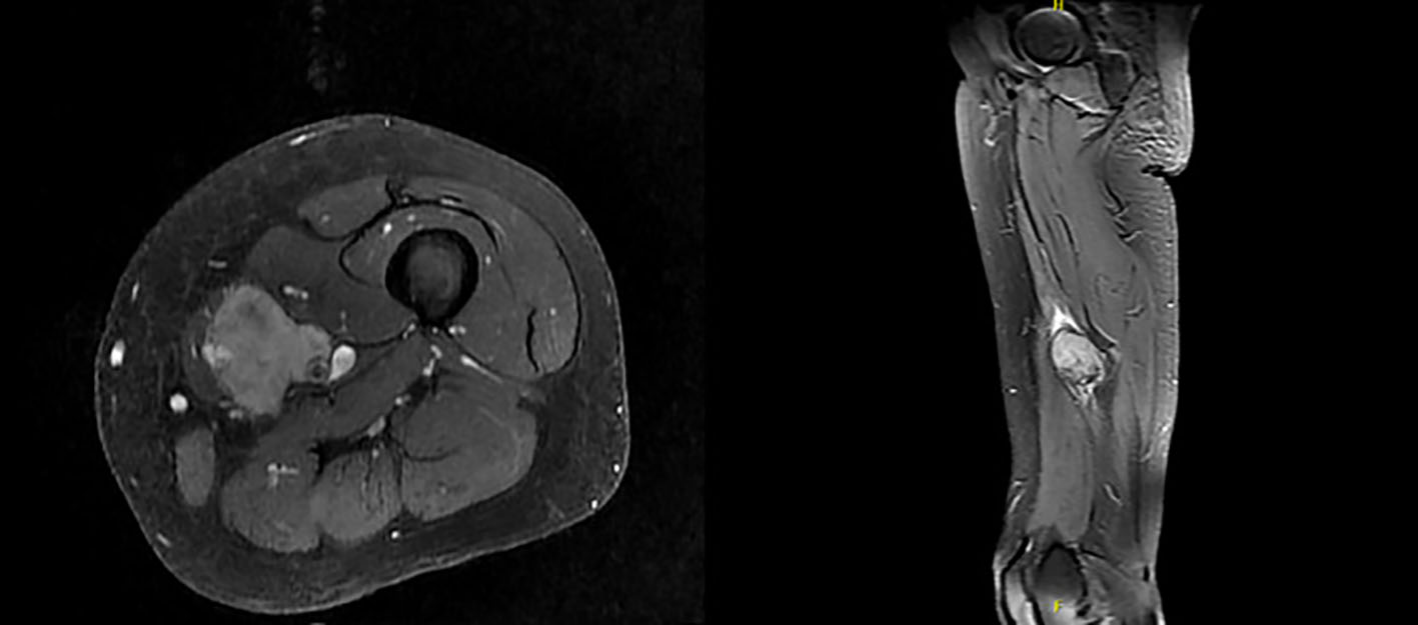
MRI of bilateral lower extremities with contrast in January 2024.
